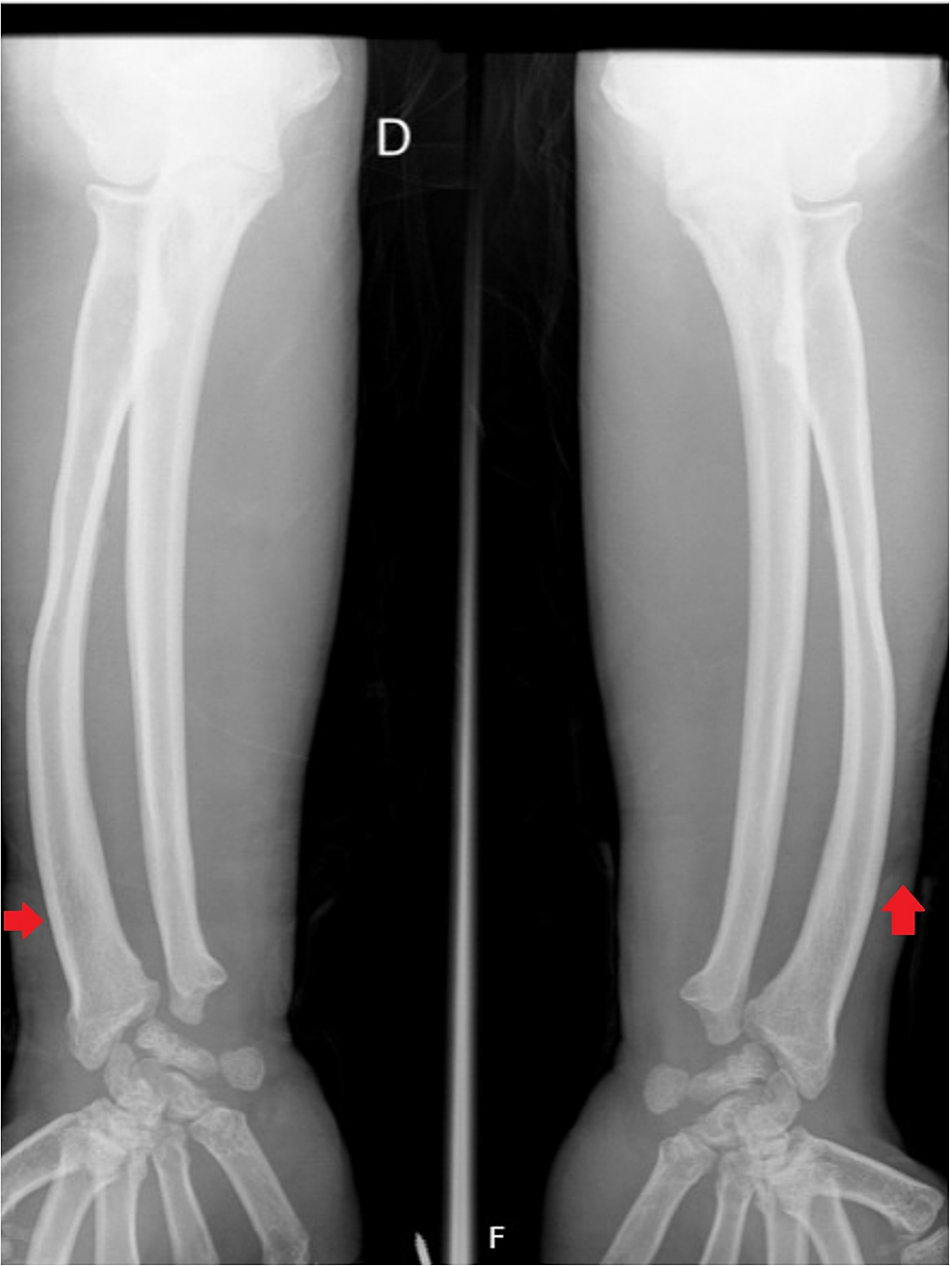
Plain X-ray of our patient's arms with dysostosis multiplex manifested as distal radius and ulna with abnormal angulation and tilting of the distal epiphyses (red arrow).
